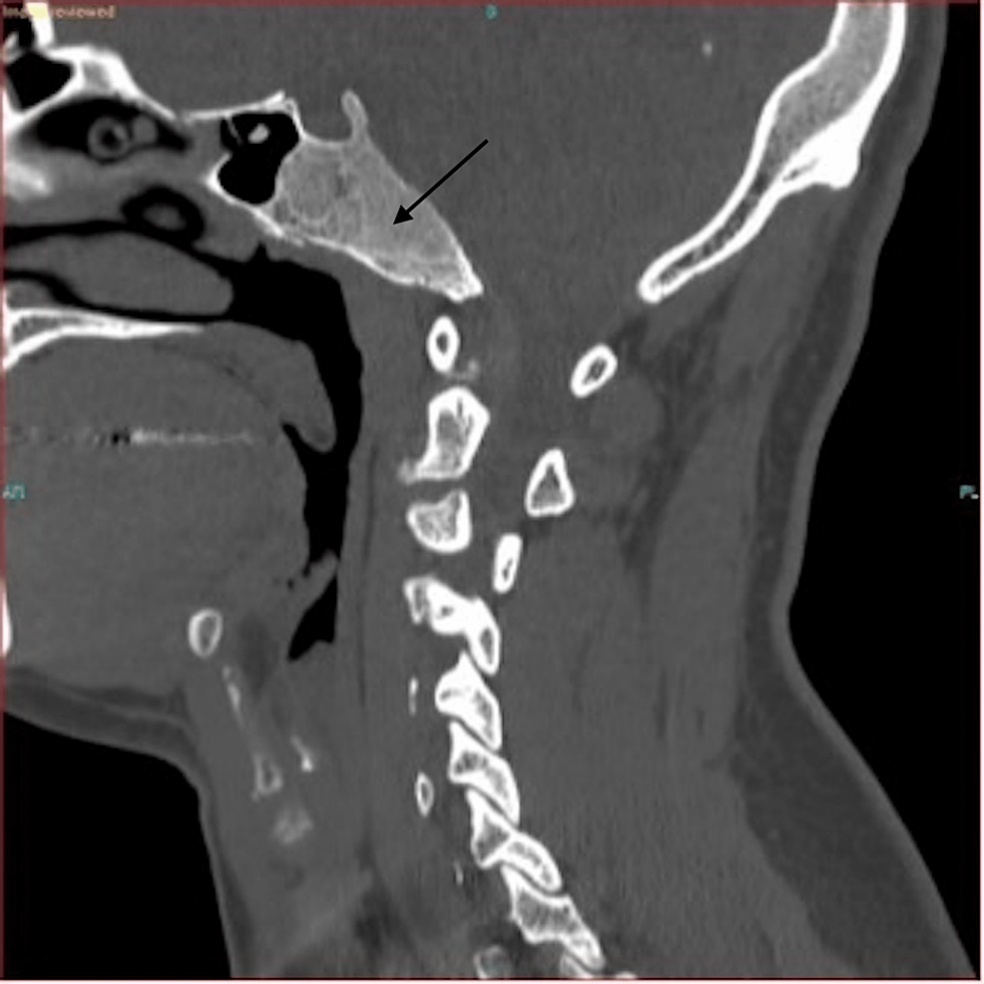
Mass in the clivus of low, hypointense signal intensity on T2-weighted image
